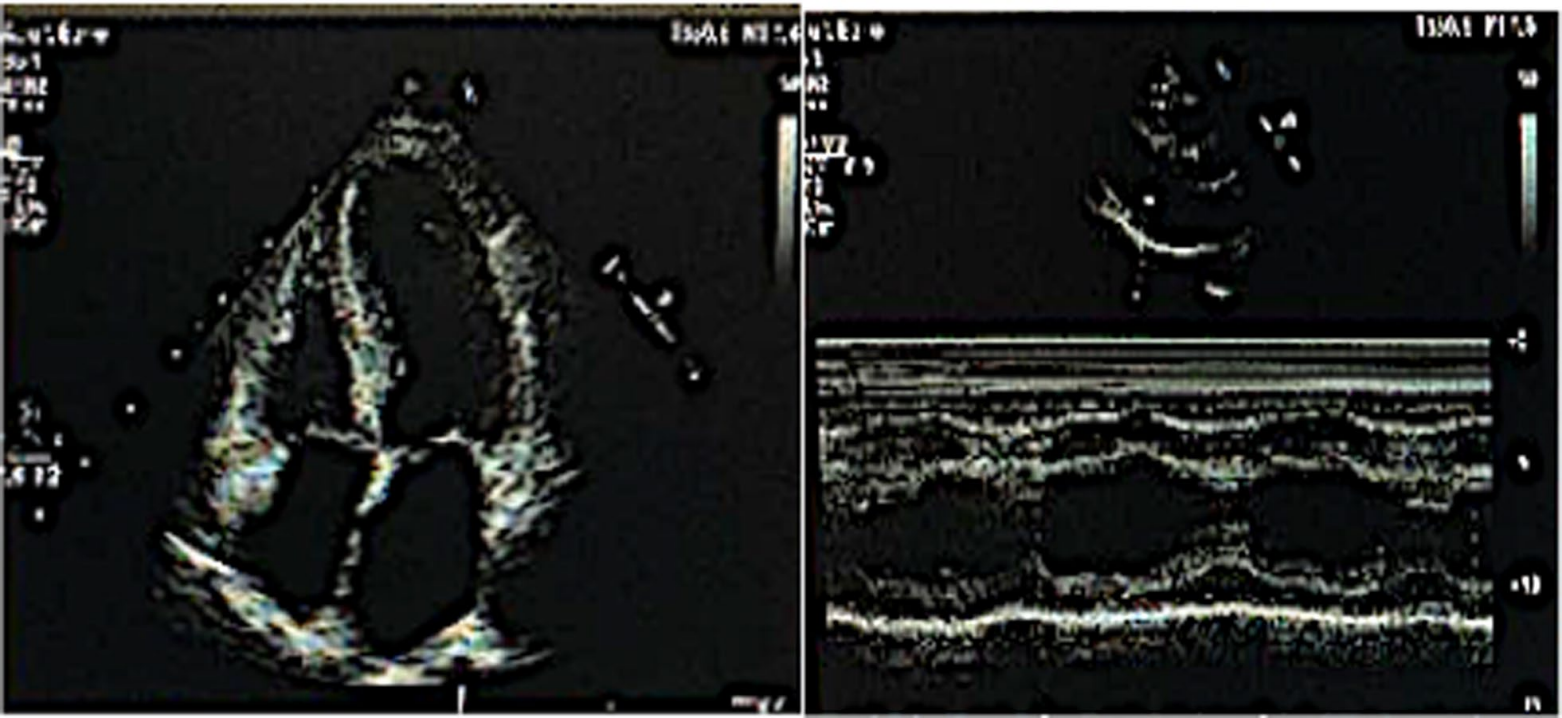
Image acquisition for colour Doppler echocardiography
